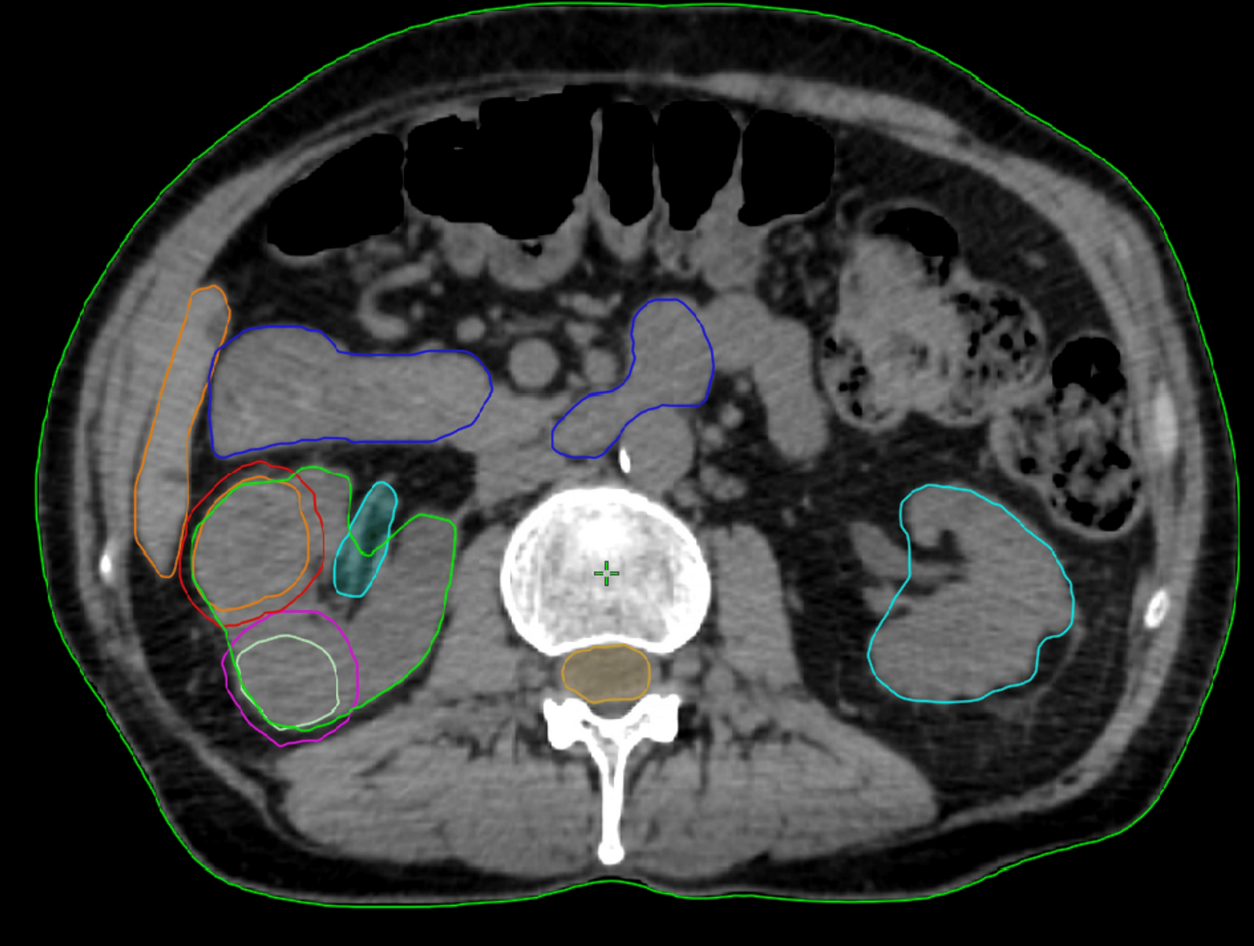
Delineation of GTVs and organs at risk on the planning CT scan GTVs, gross tumor volumes
